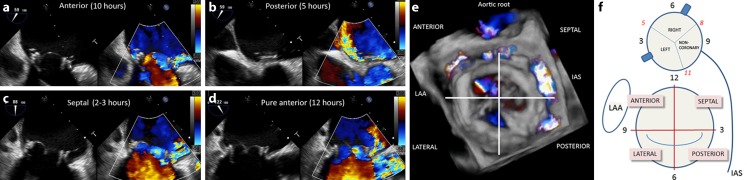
Mechanical prosthetic valve with four PVLs (a–d show different positions of leaks in 2D and colour echocardiography). To locate and assess different leaks on 2D echo needs a careful search. Instead, colour 3D-TEE makes this an easier task (e), taking into consideration the lower temporal and spatial resolution. It is necessary to refer PVL in relation to anatomic reference points (f). Taking the surgeon’s view from left atrium, leaks would be referred to in hours, as a clock, taking mitro-aortic curtain as 12 h and/or by position segments. *LAA* left atrial appendage, *IAS* interatrial septum
